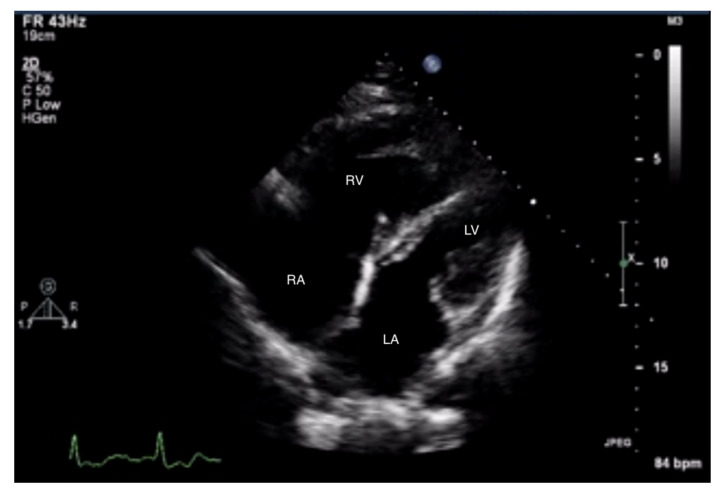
Enlarged right ventricle and right atrium. An abnormal size ratio between the right and left heart. 2D-TTE, 4CH view. 2D-TTE: two-dimensional transthoracic echocardiography; 4CH: four-chamber.
